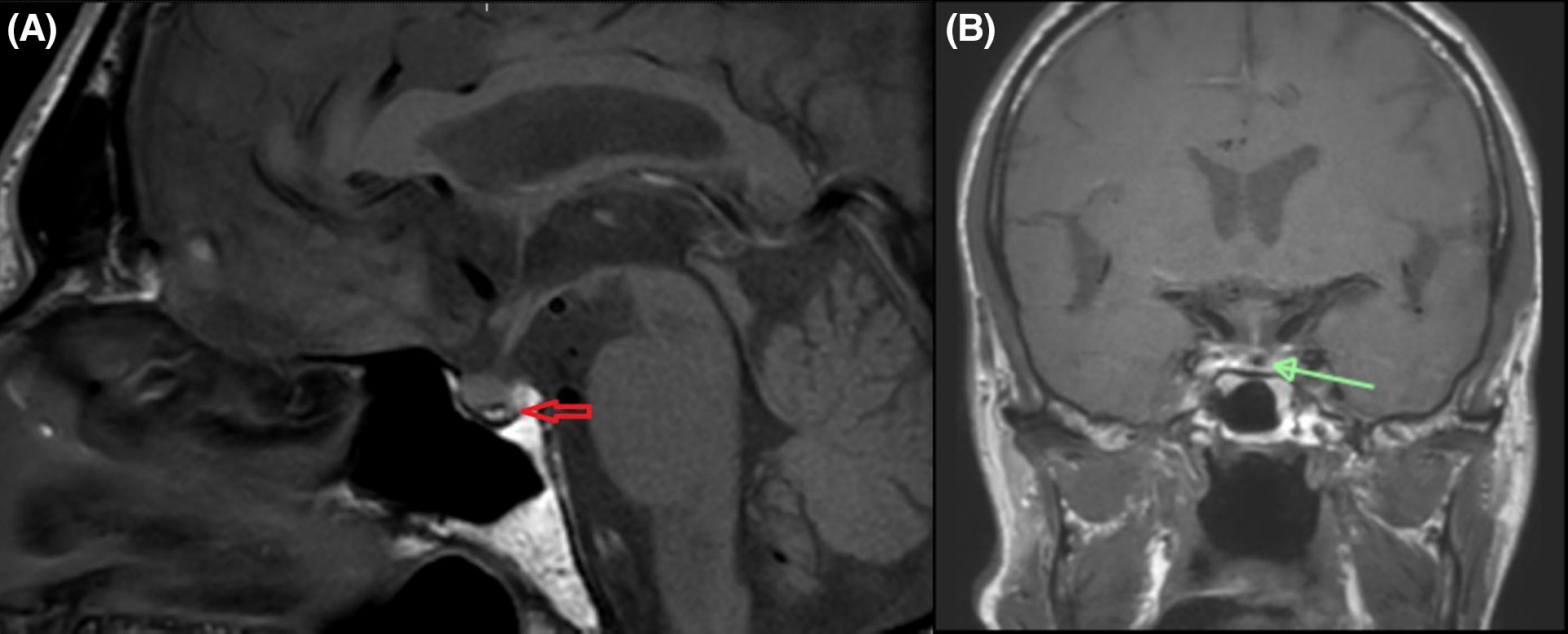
Sagittal (A) and coronal (B) T1 weighted MRI brain showing cystic lesion.
